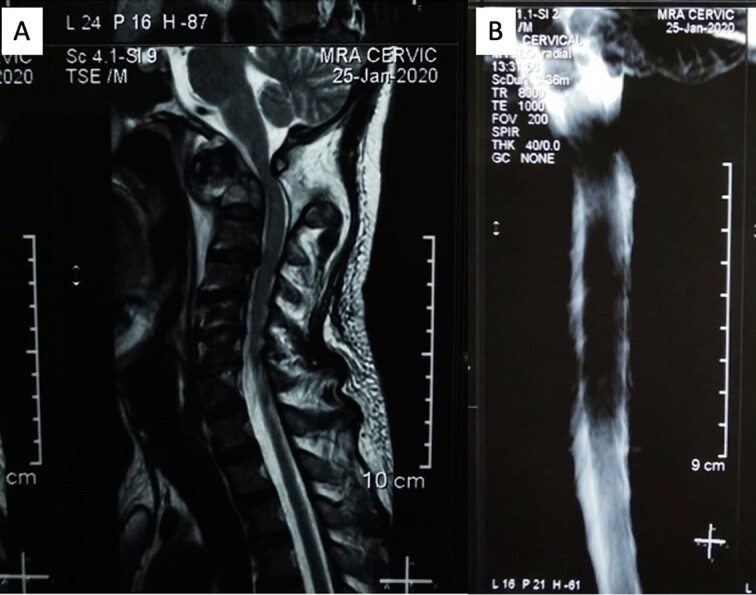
(A) Sagittal MRI showed significant compression on the occipitocervical junction due to atlantoaxial dislocation with blockage from (B) MR myelography.
